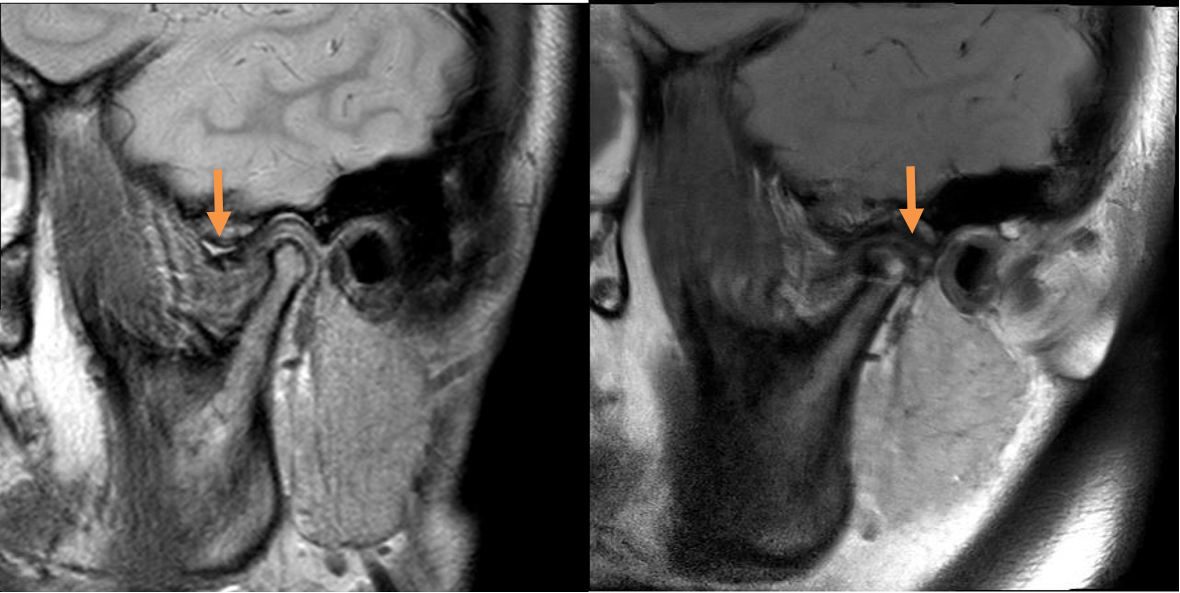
a, b Pre- and postoperative (1 year) MRI of a female patient who underwent left joint disc plication surgery. The disc position is marked with an arrow
